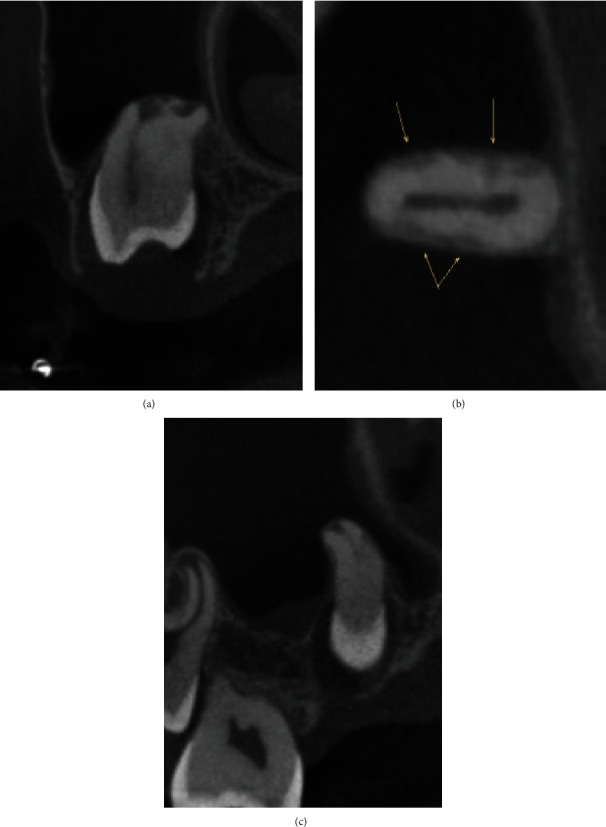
Initial CBCT: (a) coronal section, advanced ECR at apex, (b) axial section arrows indicating lateral extensions, and (c) sagittal section showing lateral extensions.
